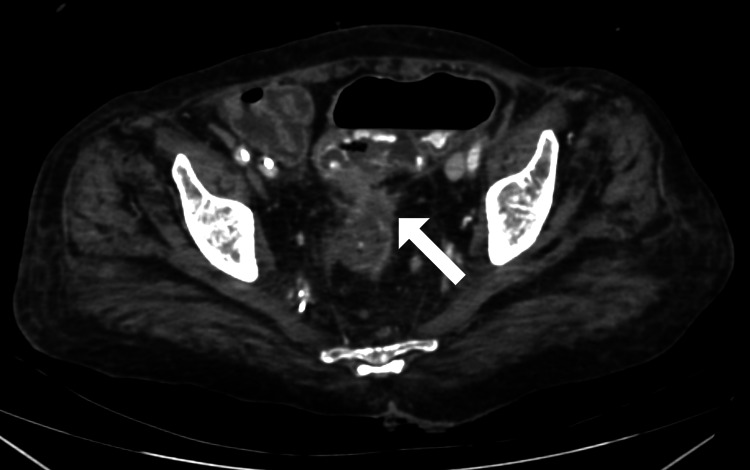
Axial post-contrast CT image of the abdomen showing irregular mural thickening and luminal narrowing involving the rectosigmoid junction
